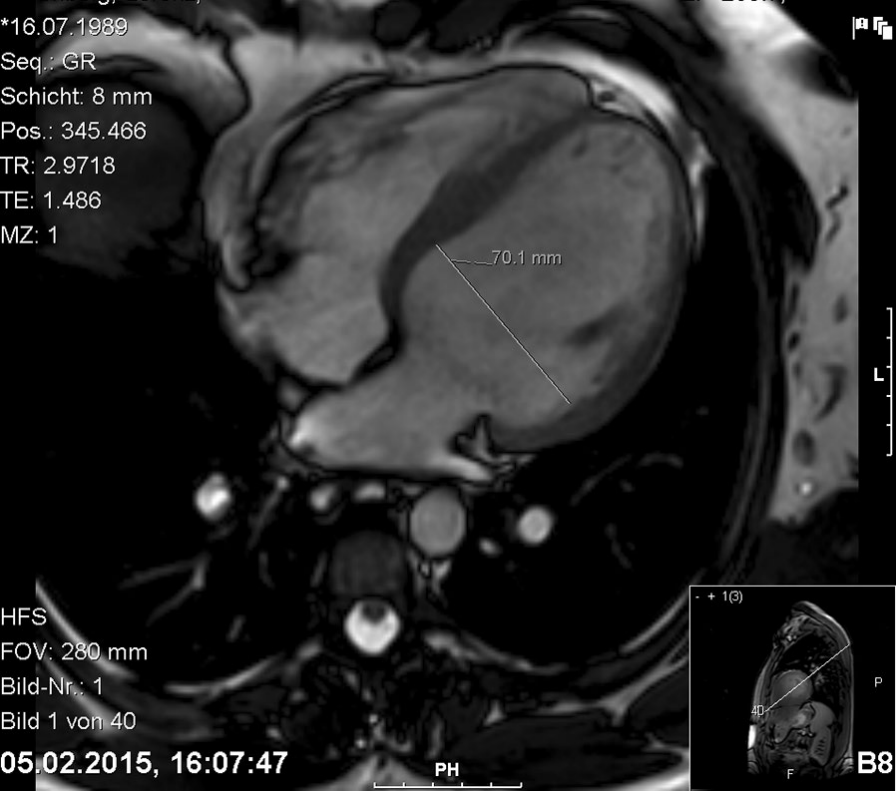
Cardiac MRI, left ventricle with a diameter of 70.1 mm
